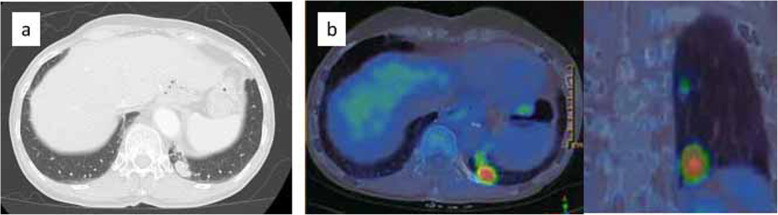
a Chest computed tomography scan shows a solitary oval pulmonary lesion, approximately 20 × 11 mm, in the left lung. b Fluorodeoxyglucose positron emission tomography-computed tomography confirms the presence of one lesion in the lung and the absence of metastases in the lymph nodes or extrapulmonary sites
